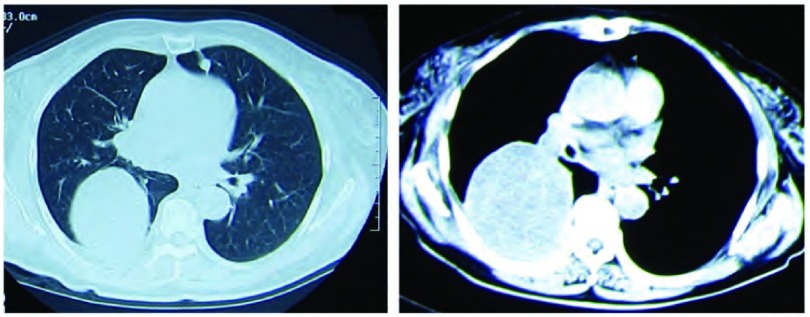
胸部增强CT示右下叶直径约6 cm的球形占位，边界光滑无分叶，呈不均匀增强。此层面CT上尚可见左舌叶小结节影。 Contrast-enhanced chest CT scan shows a spherical mass 6cm in diameter in right lower lobe. It has a smooth margin without lobulation, and is irregularly enhanced. A small nodule is also seen in left lingular lobe.
